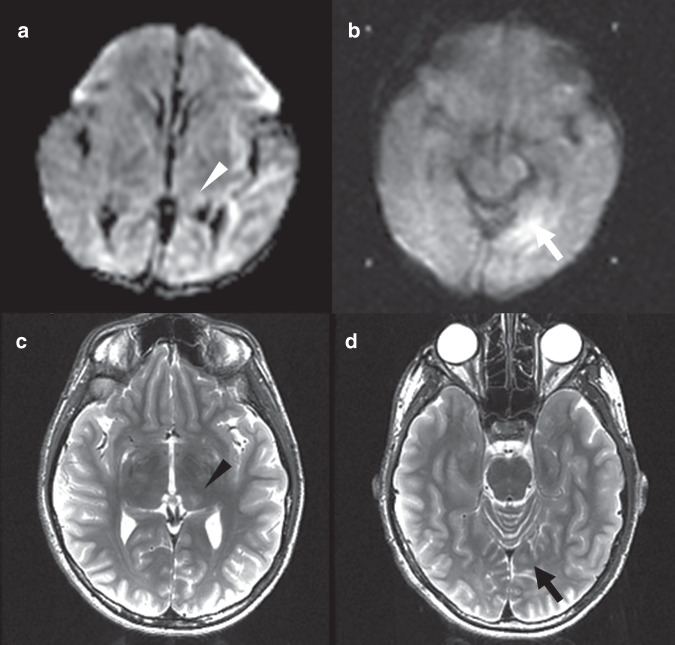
Neonatal and adolescent magnetic resonance imaging (MRI) of patient no 8. a Axial diffusion-weighted imaging of the neonatal brain demonstrates reduced diffusion in the left thalamus (white arrowhead). b Additional area of reduced diffusion in a watershed distribution in the medial left occipital lobe on neonatal MRI (white arrow). c Axial T2-weighted imaging in adolescent MRI demonstrates T2 hyperintensity and volume loss in the left thalamus (black arrowhead), corresponding to area of reduced diffusion in a. d T2 hyperintensity in the medial left occipital subcortical white matter (black arrow) corresponding to area of reduced diffusion in b.
